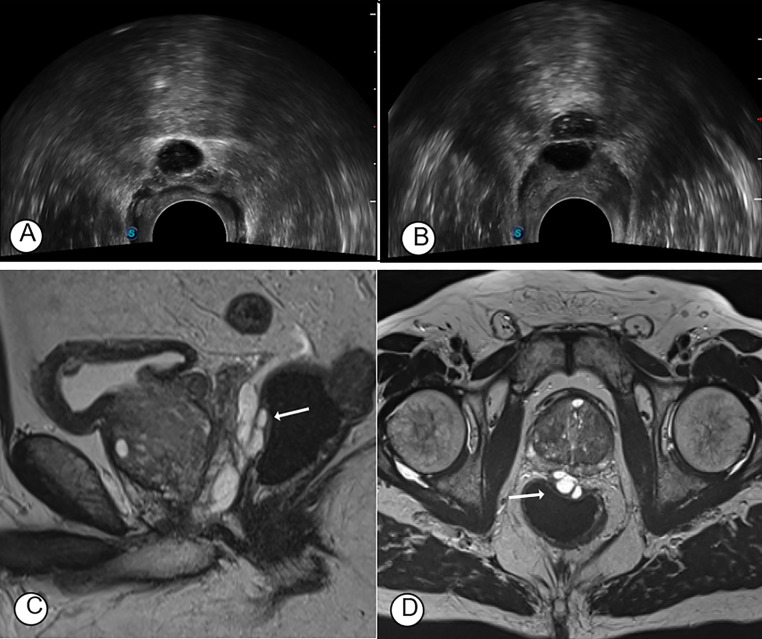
(A, B) Ultrasound imaging at the time of the procedure showing no obvious signs of the gel infiltrating the rectal wall. (C, D) Treatment planning magnetic resonance imaging scan showing potential signs of minor rectal wall infiltration (white arrow).
